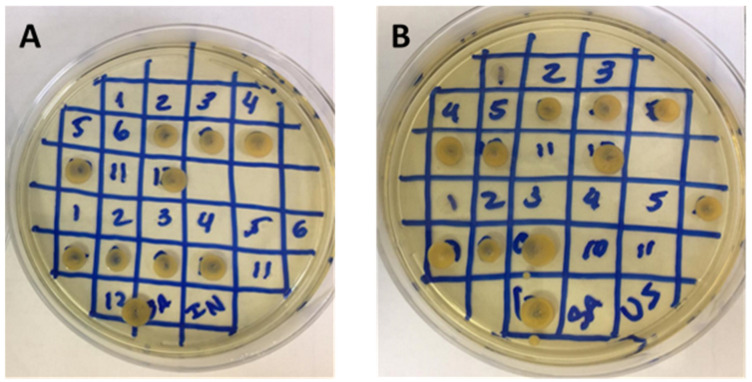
MIC assay of infusion (A) and ultrasound (B) extracts with different sample concentrations in a Petri plate. Microorganism growth was observed in diverse concentrations, as shown by the numbers. Number 12 is a positive control of microorganism growth.
